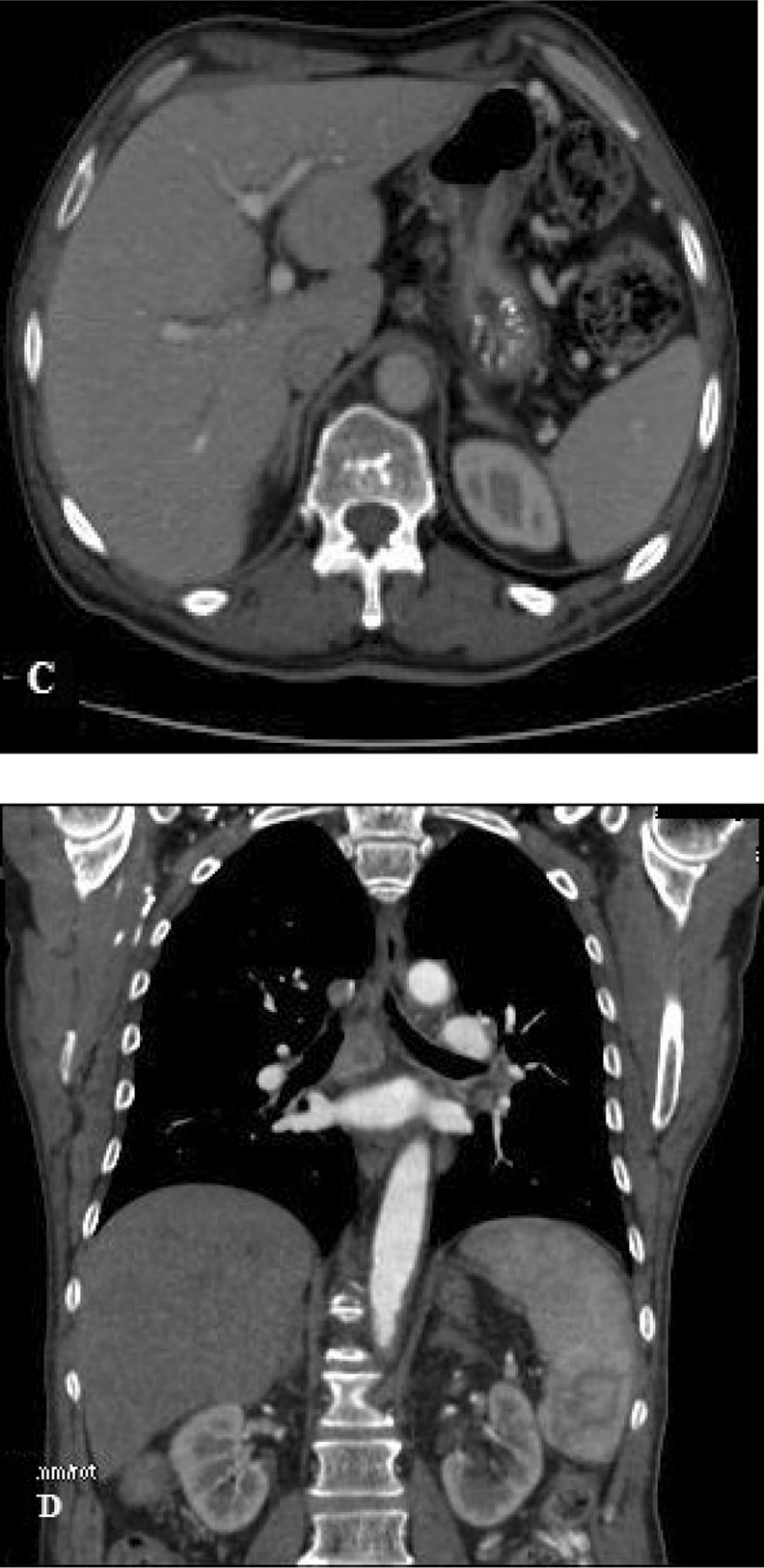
A 57-year-old man with advanced gastric cancer. C) A transverse axial contrast-enhanced CT image shows several enlarged lymph nodes along the gastrohepatic ligament. This image shows gastrohepatic ligament lymphadenopathies. Their clinical significance was uncertain. These lymph nodes were pathologically proved to be metastatic lymph nodes. D) This coronal reconstruction shows several enlarged lymphadenopathies suggesting metastatic disease which was confirmed by pathological study.
